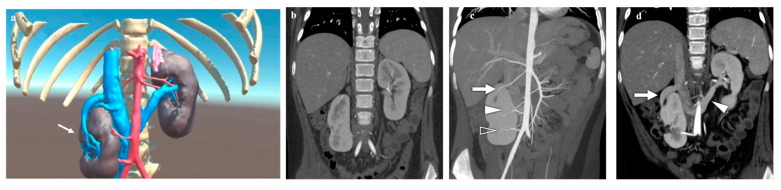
Virtual reality (VR) (a) and coronal computed tomography (CT) (b–d) images of a 10-year-old girl with a symptomatic right ptotic dysmorphic kidney. The figures show a ptotic right kidney, located inferior-medially and rotated, with normal parenchymal thickness. No urinary tract dilatation is noted. Three right renal arteries are present (→): one originates from the proximal aorta, one from the distal aorta, and one from the proximal common iliac artery; on the left, two contiguous renal arteries are depicted, and the left renal vein runs posteriorly to the aorta.
